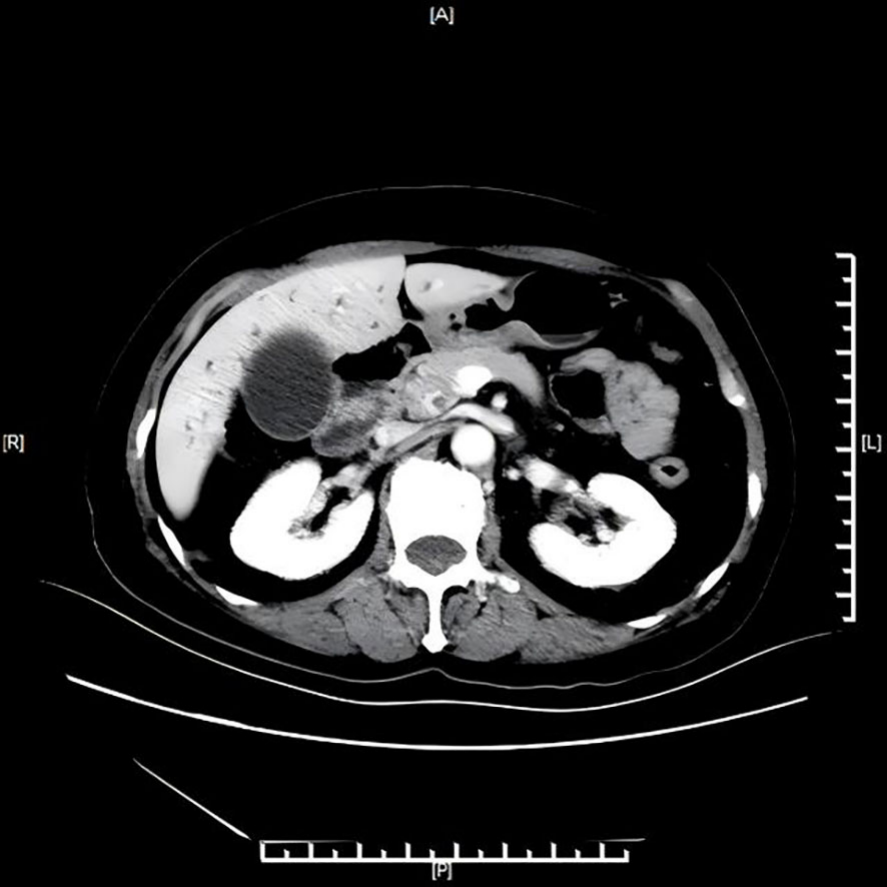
Contrast-enhanced CT showing distal biliary obstruction with ductal dilation.
